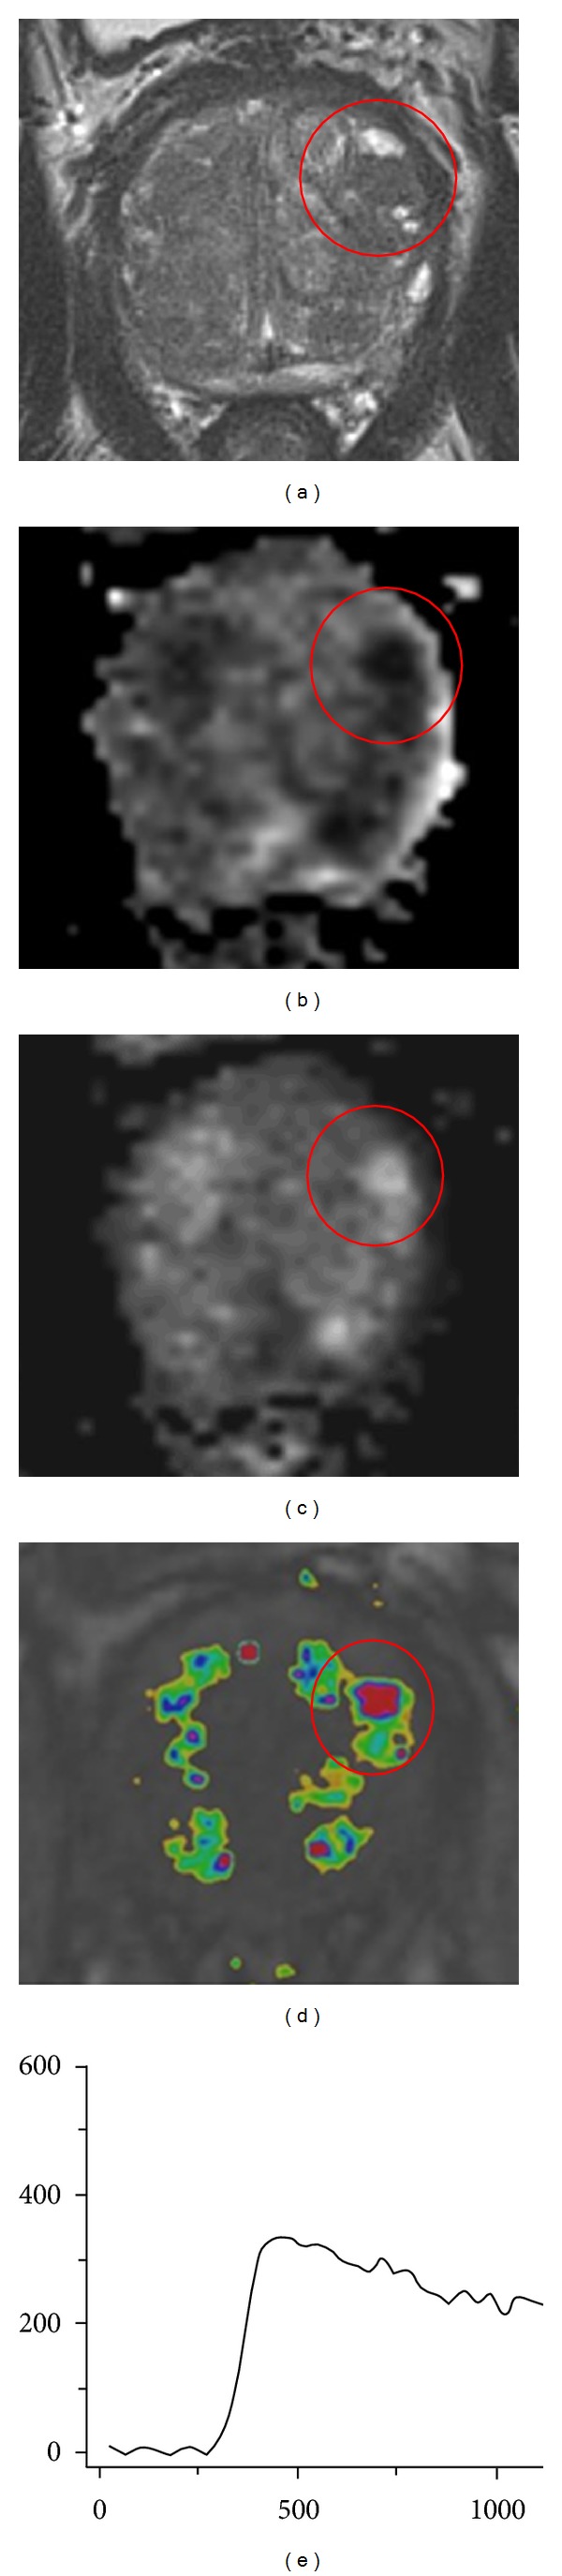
Example of multiparametric MR images of a 68-year-old male, with a PSA level of 40 ng/mL and 3 negative transrectal ultrasound guided biopsy sessions. The encircled lesion scored PI-RADS 2 on T2W image, PI-RADS 5 on DWI, and PI-RADS 4 on DCE. Because the lesion is in the transition zone, T2W is the dominant parameter, and the final PI-RADS score was PI-RADS 2. (a) Axial T2-weighted image. (b) Axial ADC map. (c) Axial DWI with *b* = 1400. (d) Axial DCE image. (e) Curve of the DCE image.
